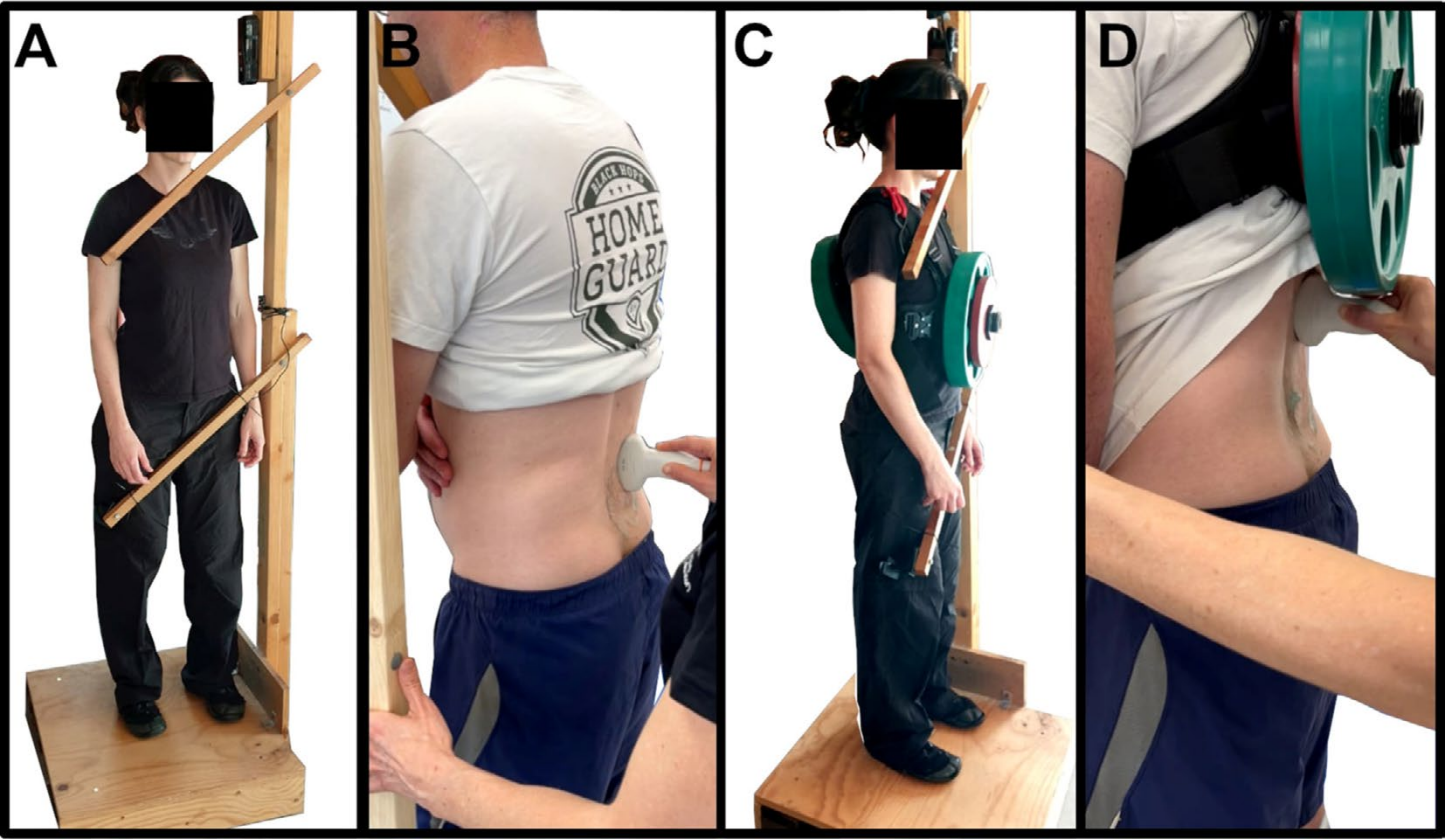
(A) Unloaded standing showing platform and position of bars. (B) Ultrasound measurement during unloaded standing. (C) Loaded standing showing platform and position of bars. (D) Ultrasound measurement during loaded standing.
